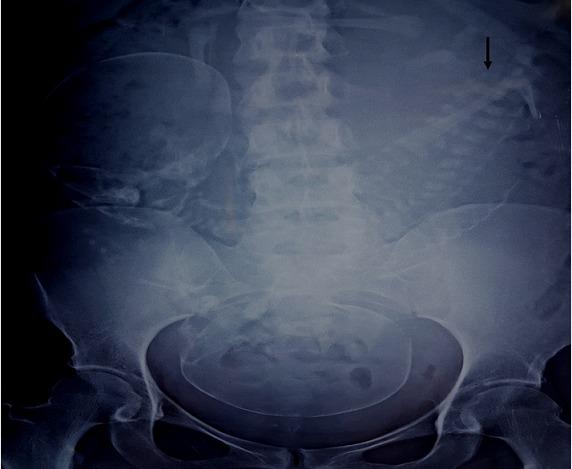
Image de la radiographie du contenu utérin montrant les deux têtes fœtales et les deux rachis fusionnant au niveau lombaire (flèche noire)
